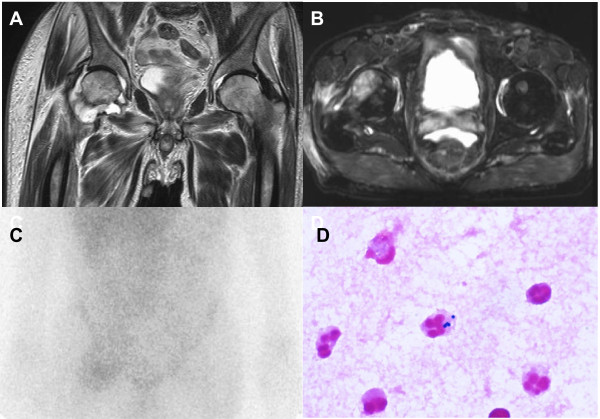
Magnetic resonance imaging of right hip joint. T2-weighted image (T2WI) of hip joint shows high-intensity area surrounding head of right femur, indicating fluid collection (A). Fat saturated T2WI shows high-intensity area on right obturator externus or adducent muscles (B) that appears as a hotspot on gallium scintigram (C). Synovial fluid contains abundant leukocytes that are phagocytically engulfed by gram-positive cocci (D).
